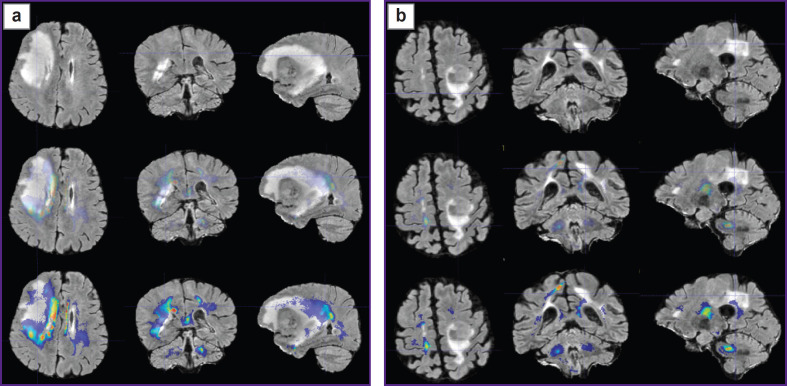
Plotting the maps of value gradients of kurtosis anisotropy (KA) in patient with glioblastoma (а) and patient with metastasis (b) Upper row — T2-FLAIR images in three projections; middle row — comparison of T2-FLAIR with KA parametric map (semitransparent for better contour visualization of the changed MR signal by T2-FLAIR); lower row — comparison of T2- FLAIR with KA parametric map (in full color, where red and yellow tones show the zone of greatest parameter values)
